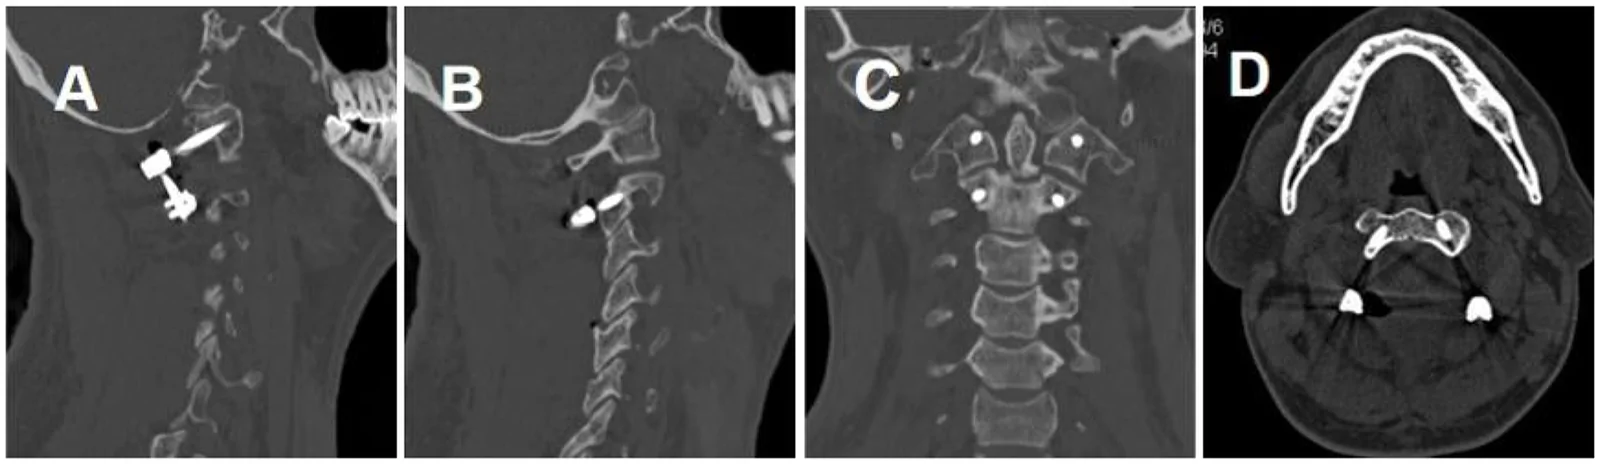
Postoperative imaging studies. (A, B) Sagittal, (C) coronal, and (D) transverse views of the three-dimensional computed tomography (3D-CT) reconstruction of the cervical spine.
